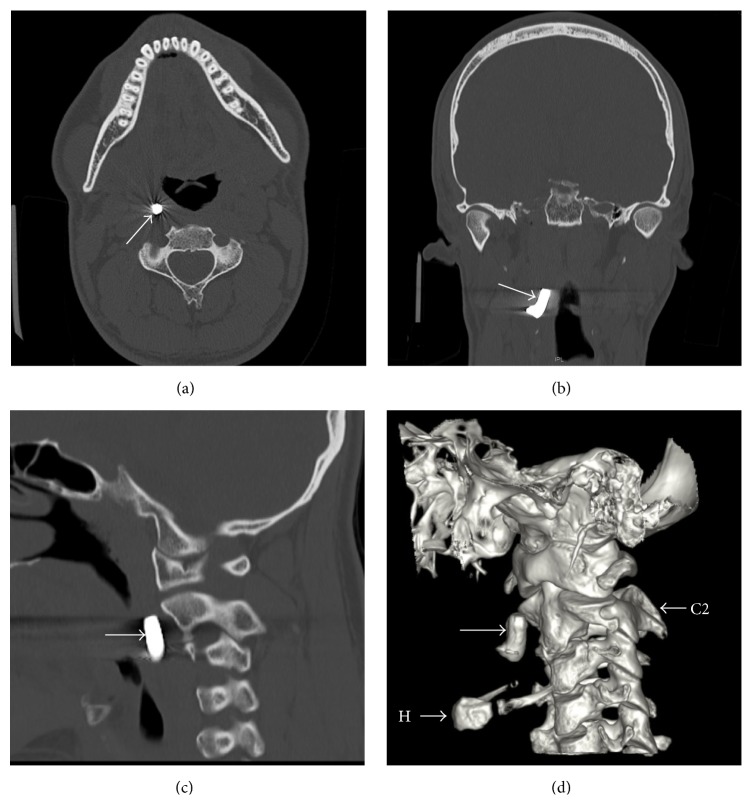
Axial (a), coronal (b), and sagittal (c) CT scans of the head and neck region showing the projectile in the parapharyngeal space, just ventrally to the longus colli muscle and medially to the carotid sheath. (d) A three-dimensional reconstruction (left lateral view). Arrow: projectile, H: hyoid, and C2: vertebra C2.
